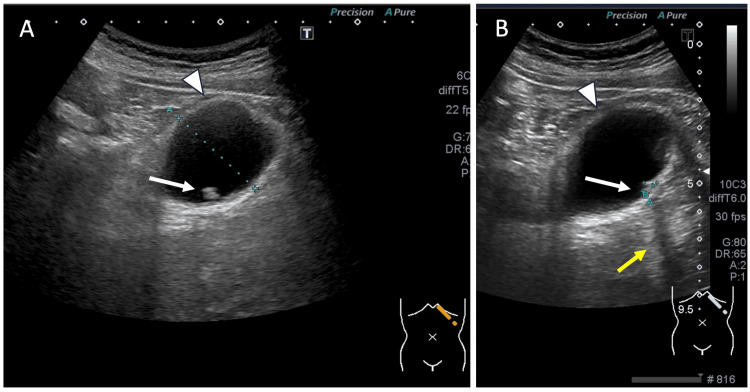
Abdominal ultrasound. A: An ultrasound image shows multiple hyperechoic lesions (white arrow) within the distended gallbladder (arrowhead). B: Another view from the same examination demonstrates posterior acoustic shadowing (yellow arrow), a characteristic feature of gallstones, although the calculus itself is partially obscured by the measurement cursor. Based on these findings, a clinical diagnosis of cholelithiasis was made (white arrow: multiple hyperechoic lesions, arrowhead: gallbladder).
